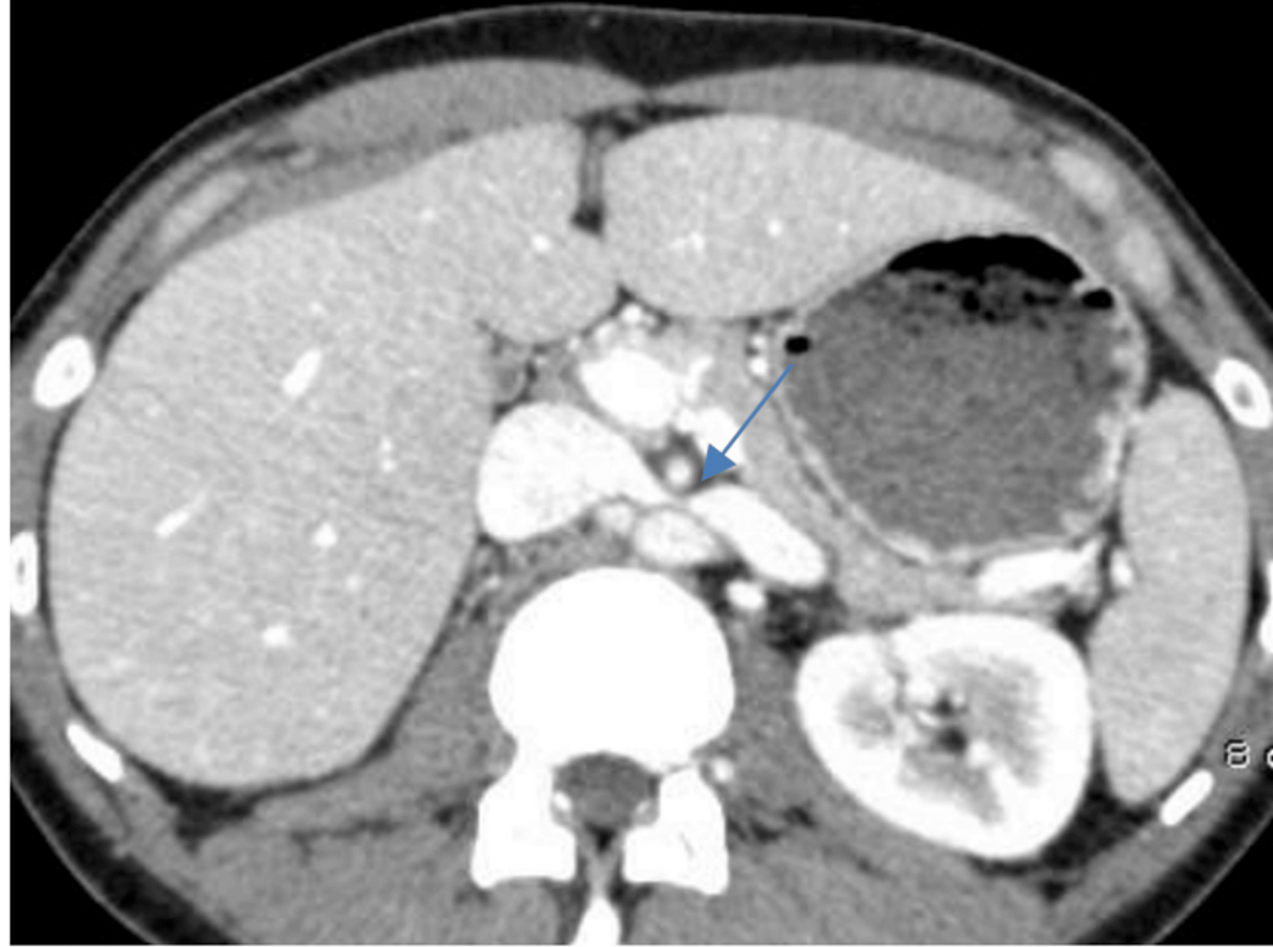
Axial section of an abdominal-pelvic CT scan after contrast injection. Axial section of an abdominal-pelvic CT scan after contrast injection showing a narrowing of the left renal vein in the aorto-mesenteric fork, forming the "beak sign" (arrow), with the ratio of the hilum portion diameter to the aorto-mesenteric portion of the left renal vein measuring 7.4 (>4.9).
